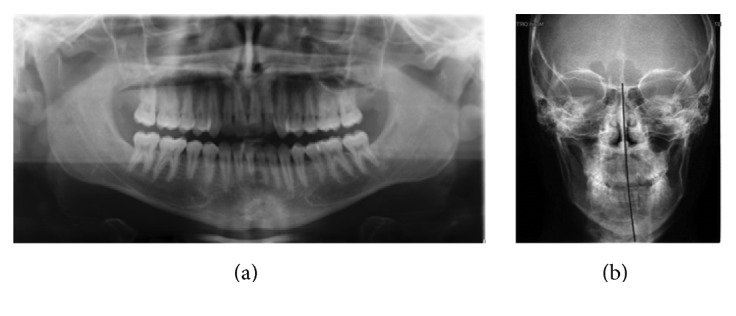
Preoperative radiological evaluation. (a) Orthopantomograph; (b) teleradiography of the skull in a posteroanterior projection.
